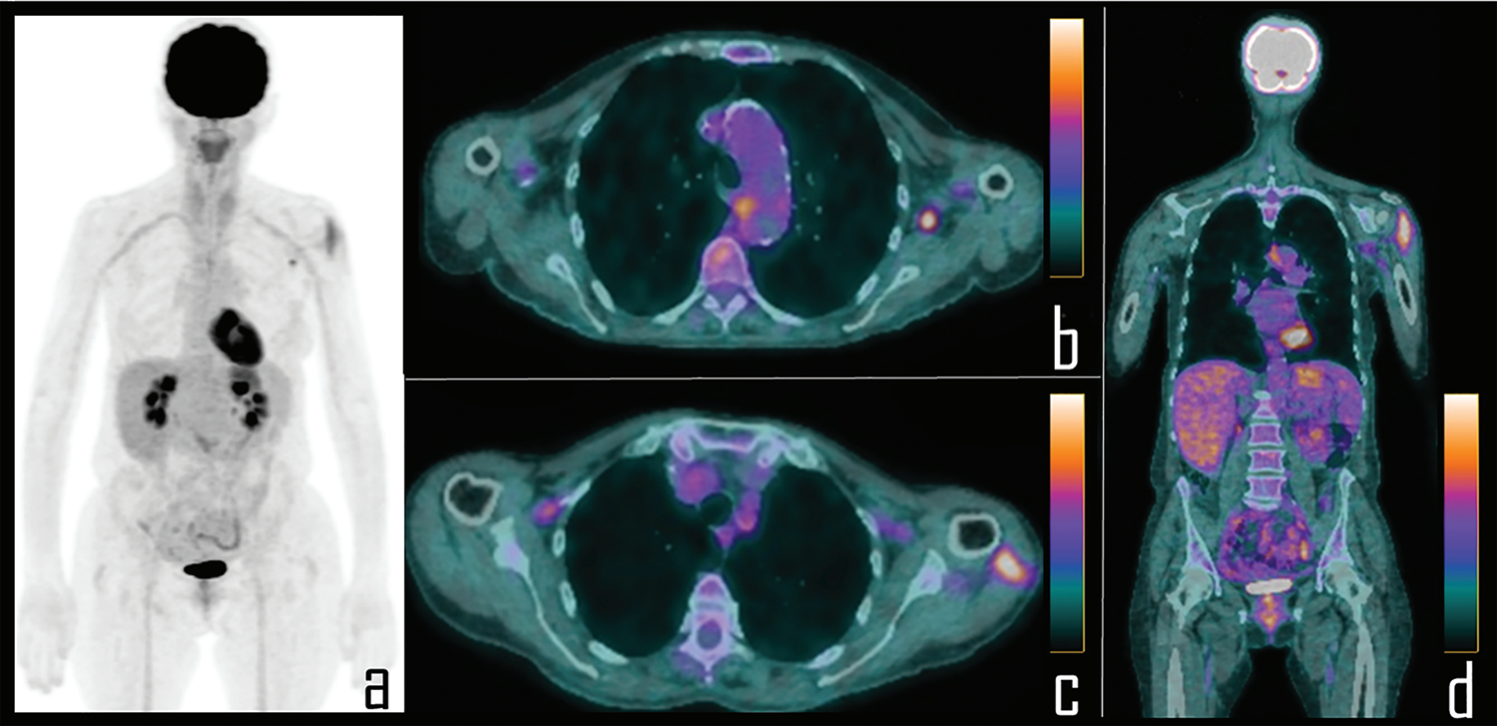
A 56-year-old female patient underwent 2-[^18^F]FDG PET/CT during follow up of colon cancer. PET *Maximum Intensity Projection* (a) was negative for disease relapse but showed focal area of uptake in the left axilla and diffuse tracer uptake in the ipsilateral arm. Axial PET/CT views better display the uptake respectively associated to a single axillary lymph node (b) and the ipsilateral deltoid muscle (c), which was the site of the second COVID-19 vaccine administration, occurred 8 days before the scan. Coronal PET/CT view (d) better displays diffuse uptake in the left deltoid muscle.
